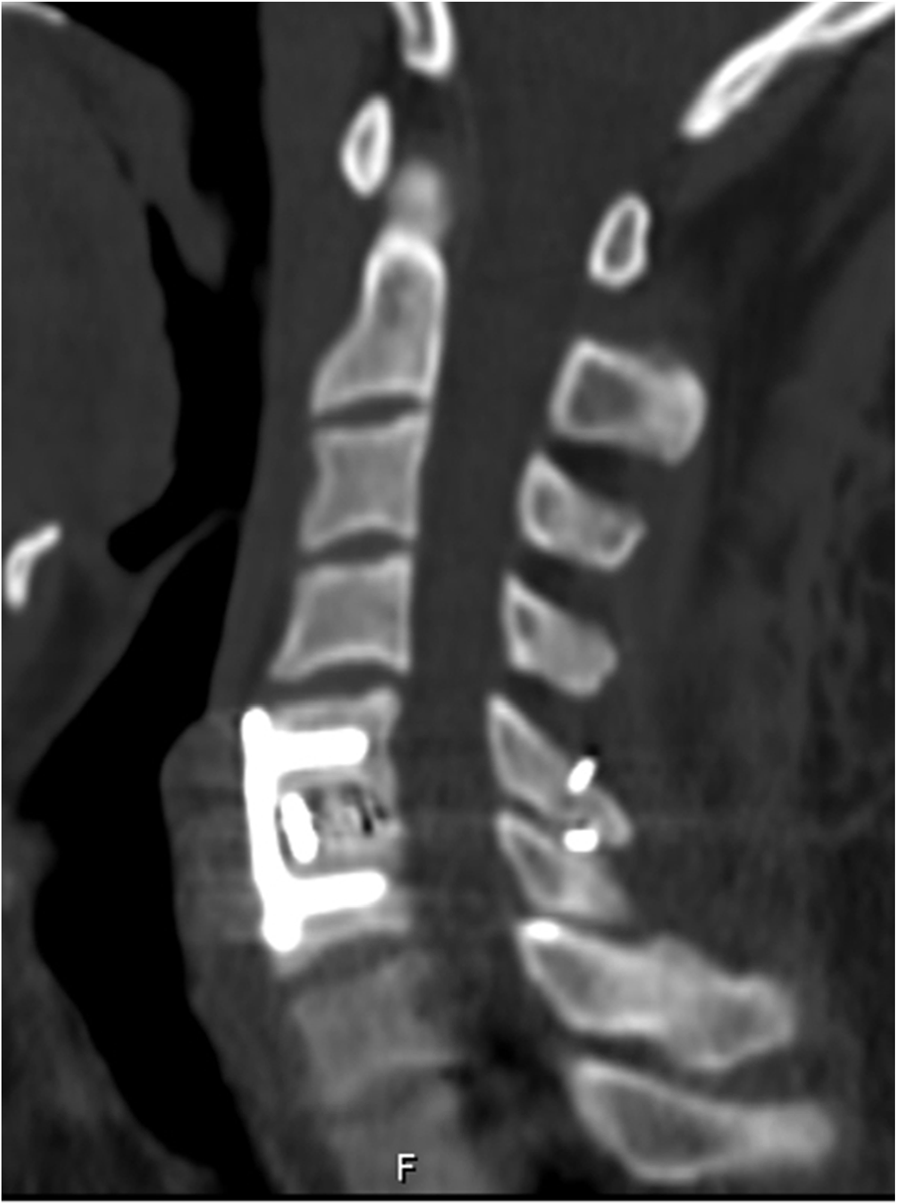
Computed tomography scan at the 24-month follow-up: excellent bony consolidation
